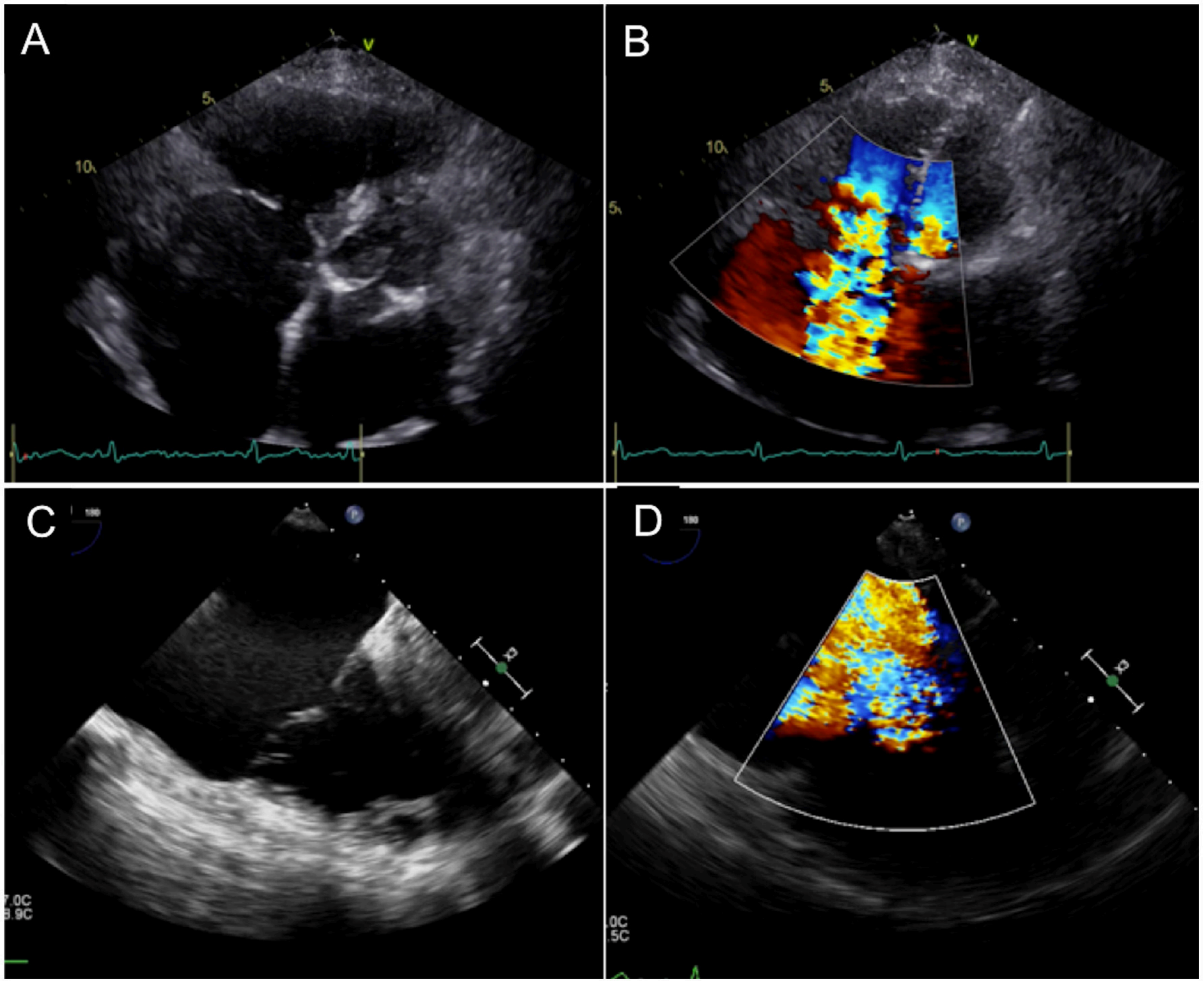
Echocardiographic Evaluation of Severe Tricuspid Regurgitation and its etiology. (A,B) Extreme tethering and annular dilatation with loss of coaptation, resulting in severe valvular regurgitation. (C,D) Large posterior leaflet prolapse with severe eccentric regurgitant jet.
